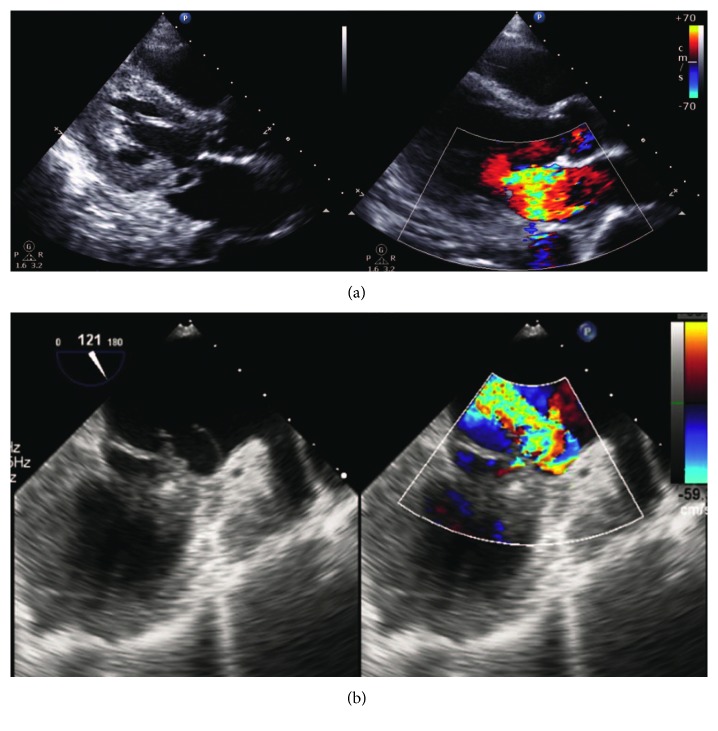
(a) In parasternal long-axis view, wall motion was normokinetic and ejection fraction was over 60%. Posterior leaflet of mitral valve (P1) had a valvular aneurysm and perforation of the valvular aneurysm. Severe mitral regurgitation is shown in color Doppler echo imaging. (b) Transesophageal echocardiography showed that posterior leaflet of mitral valve (P1) had a valvular aneurysm, with perforation of the valvular aneurysm and severe mitral regurgitation, but did not have vegetation or abscess of the mitral valve annulus.
